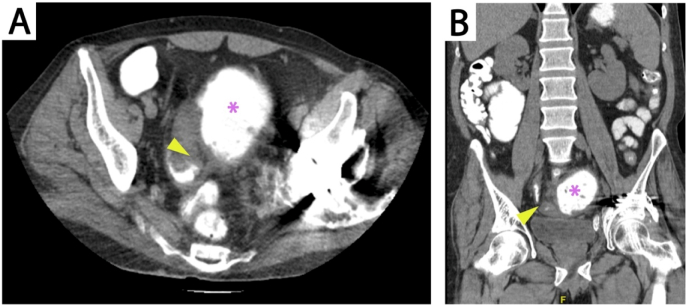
Abdominal oral contrast CT showing a transition zone at the terminal ilium (arrow) and proximal bowel dilatation (asterisks) in axial (A) and coronal (B) views.
